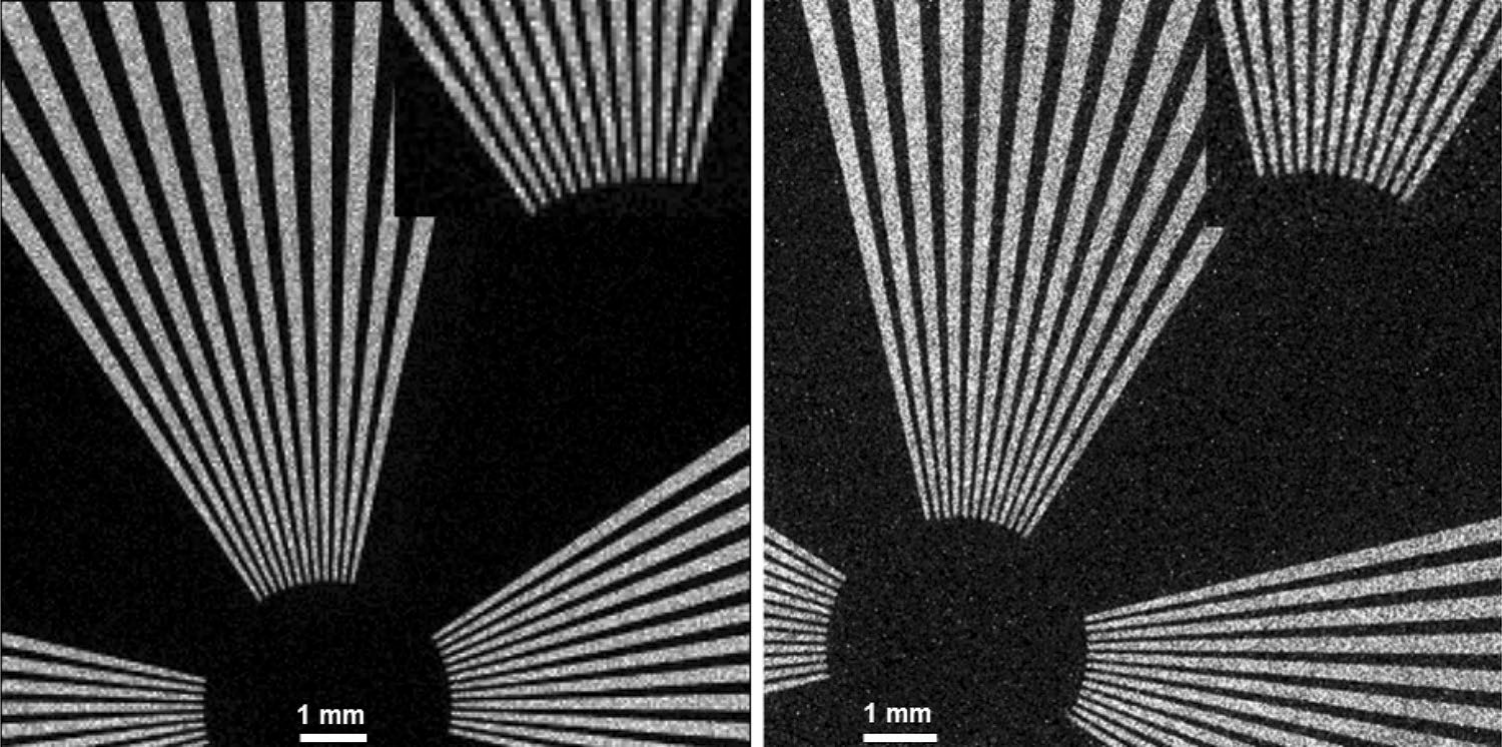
X-ray resolution target images using (left) Timepix camera with a Si sensor, and (right) PEALPB camera. The insets in the picture show the magnified area of the highest resolution end of the resolution target. The innermost lines have a Nyquist frequency of 10 l p/mm.
